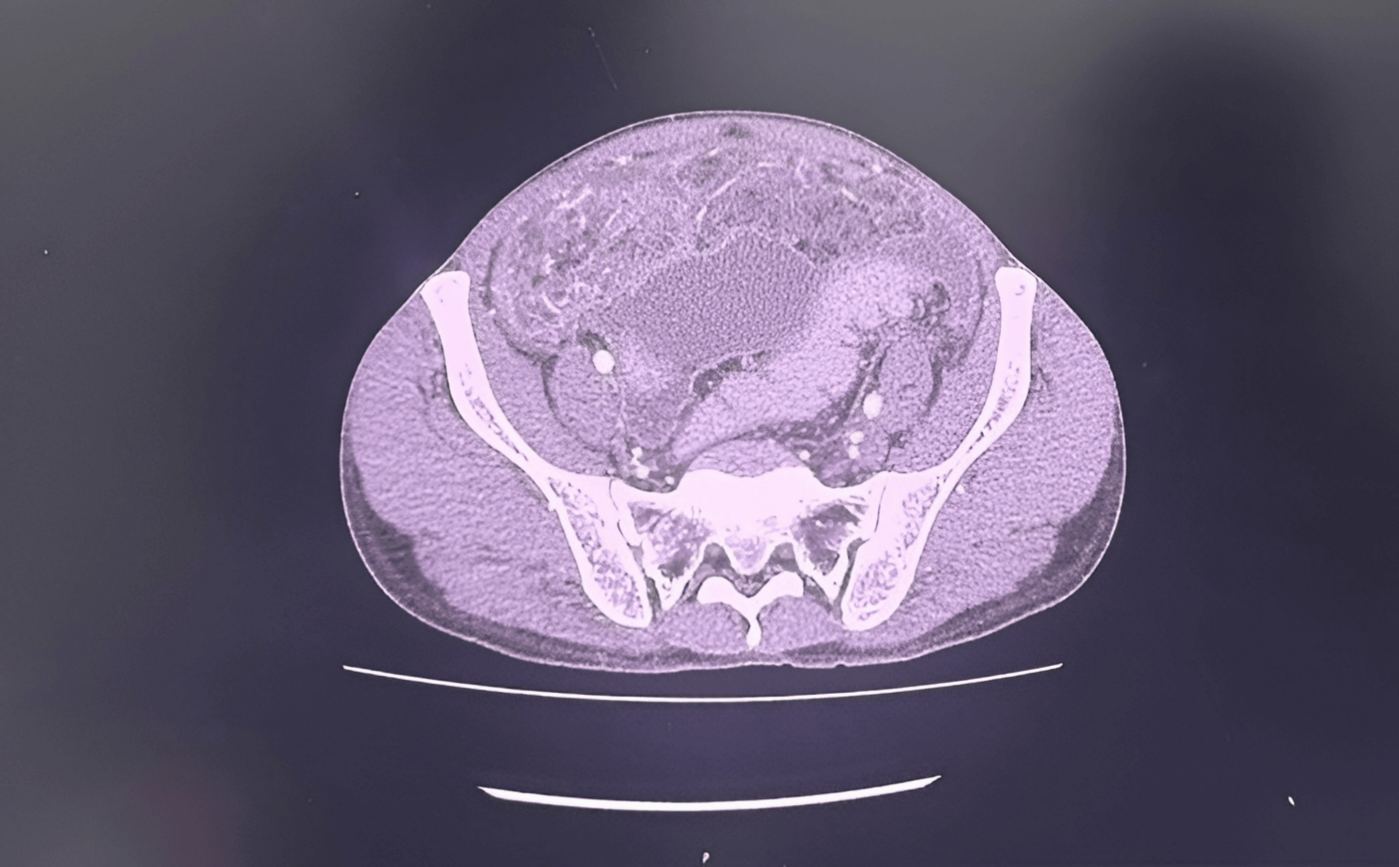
Contrast-enhanced computed tomography of the abdomen demonstrating omental thickening.
